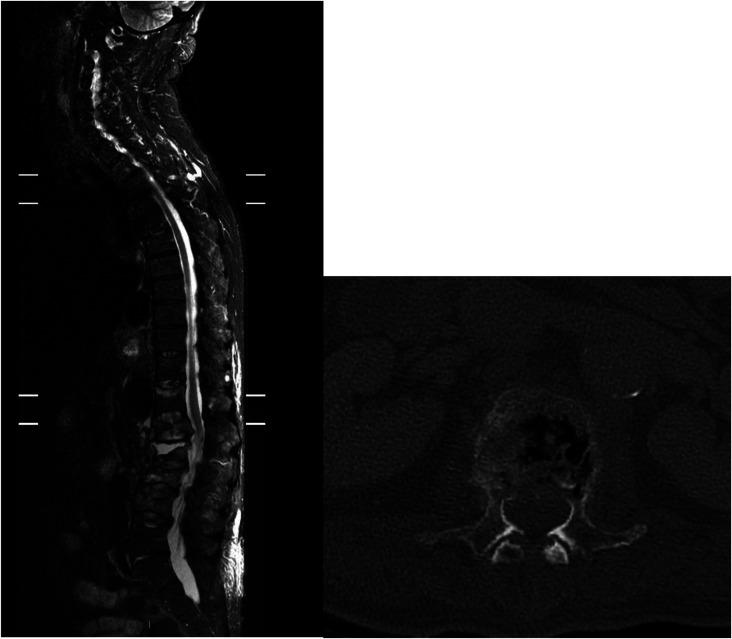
Left image – pre operative sagittal MRI STIR showing vertebral compression fractures of T12–L4 caused by myeloma infiltration. Right image – Pre-operative Axial CT scan of L2 showing a typical posterior wall defect.
